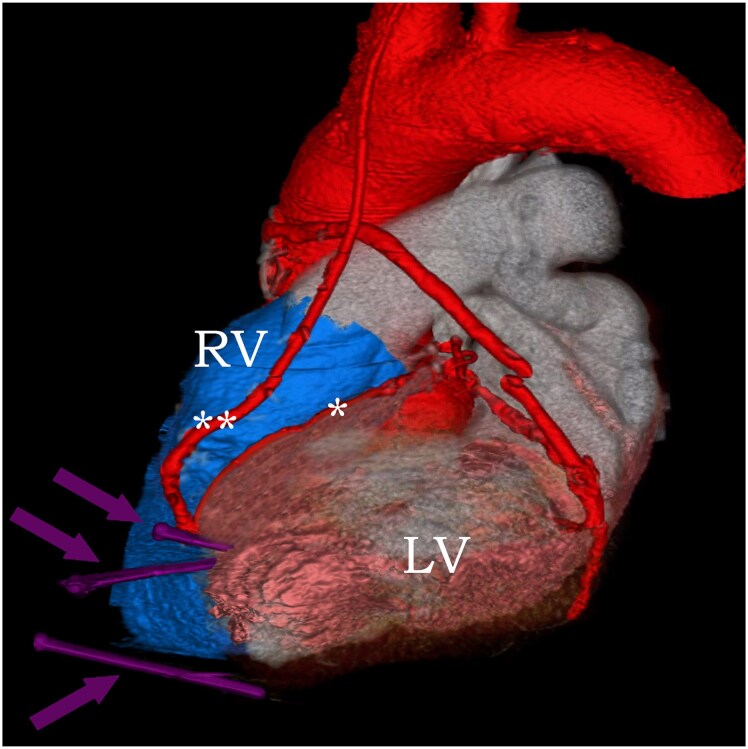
3D computed tomographic construction of the heart. Three nails are seen (purple arrows), two of them penetrate through the heart and the third one is adjacent to the inferior wall. The left anterior descending (LAD) artery (*) travels between the left ventricle (LV) and right ventricle (RV) and the left internal mammary arterial graft () is anastomosed to it. The two nails penetrate the heart close to both the left anterior descending artery and left internal mammary artery.
